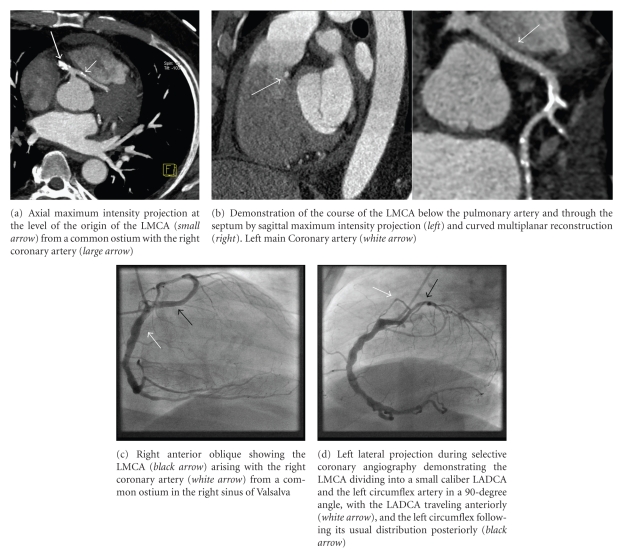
Transseptal course of the right-sided left main coronary artery by CTA and selective coronary angiography.
